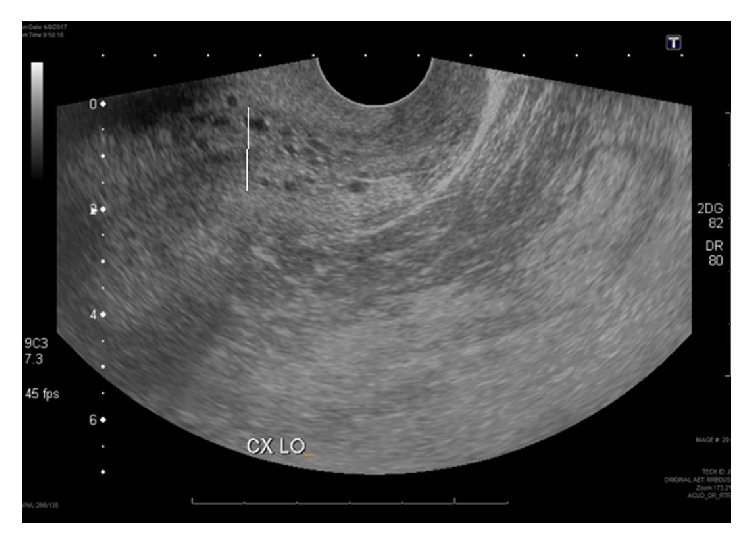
Ultrasound imaging of uterus demonstrating endometrial lining measuring 20 mm containing small cystic appearing areas.
